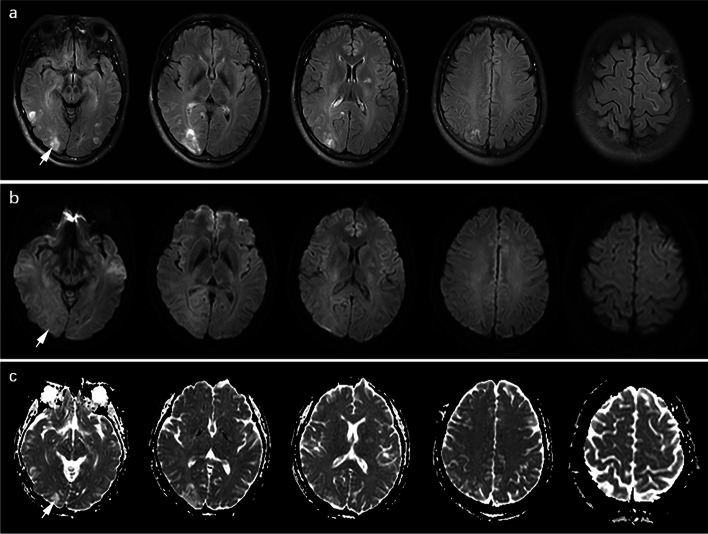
Cranial MRI of a 31-year-old woman with preeclampsia with PRES: a axial T2W FLAIR-SPIR, b axial DWI with b value of 1000 s/mm^2^, and c axial ADC map. SBE was evaluated according to the distribution and degree of abnormal edema signal, which presented as heterogeneous hyperintense signals (arrow) on T2W FLAIR-SPIR (a). Large area fusions of abnormal edema signals were shown in the right occipital and temporal lobes; and multiple flecks, patches, or nodules of abnormal signals without fusion were seen in the left basal ganglia, right parietal lobe, left occipital, temporal and frontal lobes. The SBE of typical regions, atypical regions, and all regions in this patient were 7, 9, and 16, respectively. The abnormal edema signals presented as isointense signals (arrow) on DWI (b) and as hyperintense signals (arrow) on ADC map (c)
